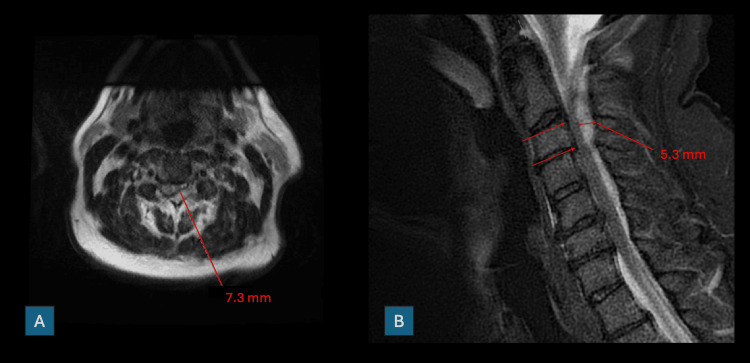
Key images from the emergent MRI brain, which identify the extent of the spontaneous cervical epidural hematoma (SCEH). (A) Axial view. (B) Sagittal view.
